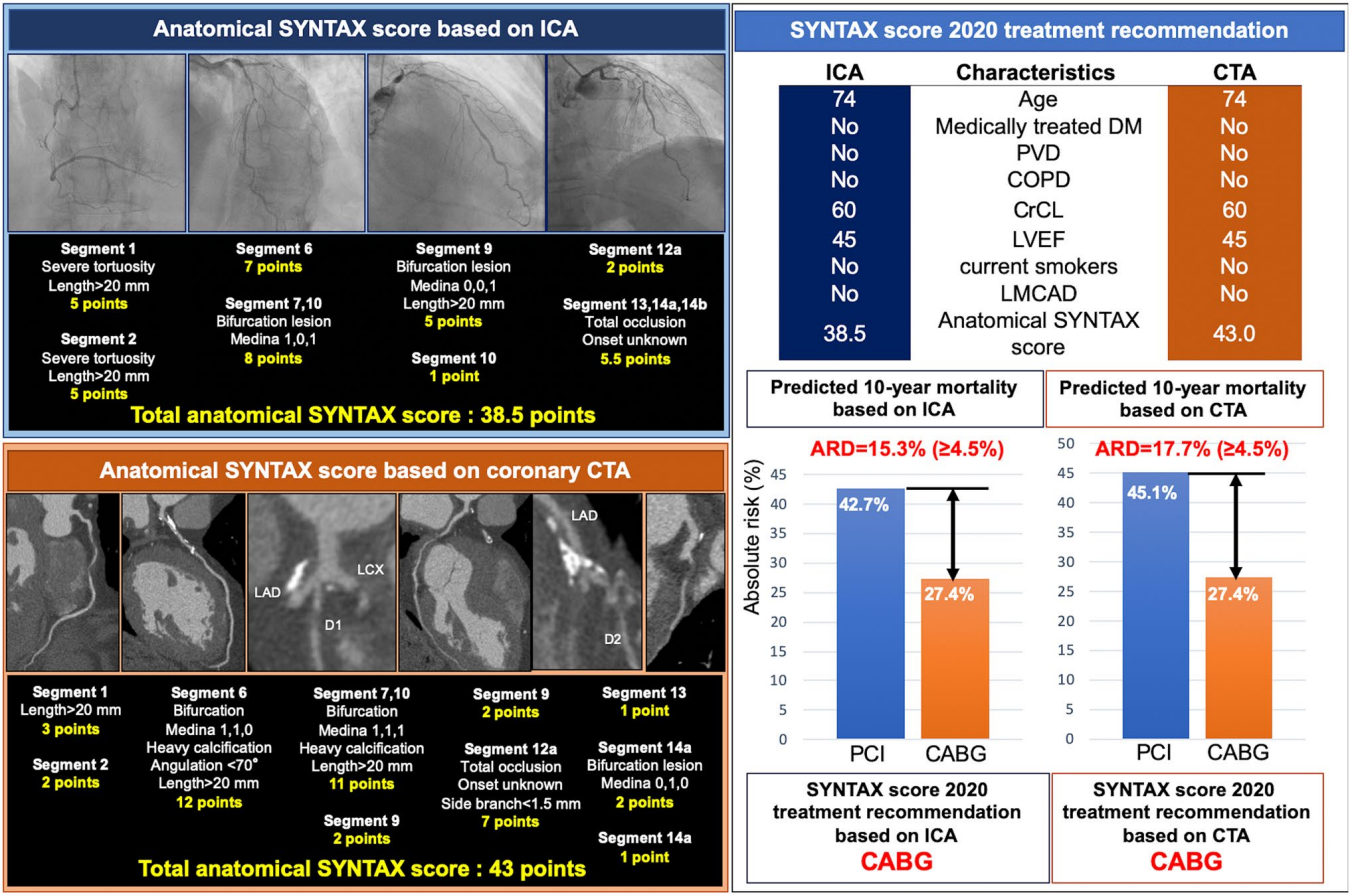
Representative case of SYNTAX score 2020 calculation. A 74-year-old man with creatinine clearance of 60 ml/min and LVEF of 45%. The anatomical SYNTAX score derived from ICA is 38.5, and from CCTA is 43.0. Predicted 10-year all-cause mortality based on ICA with PCI and CABG are estimated to be 42.7% and 27.4%, respectively. Alternatively, predicted 10-year mortality with PCI and CABG based on CCTA are estimated to be 45.1% and 27.4% respectively. Individual ARD was estimated to be 17.7% on ICA (42.7% [all-cause mortality with PCI] − 27.4% [all-cause mortality with CABG]) and 15.3% on CCTA (45.1–27.4%). Based on these findings, CABG is recommended by both ICA and CCTA. *ARD* absolute risk difference, *CABG* coronary artery bypass graft, *CrCL* creatinine clearance, *CCTA* coronary computed tomography angiography, *COPD* chronic obstructive pulmonary disease, *DM* diabetes mellitus, *ICA* invasive coronary angiography, *LMCAD* left main coronary artery disease, *LVEF* left ventricular ejection fraction, *PCI* percutaneous coronary intervention, *PVD* peripheral vascular disease
